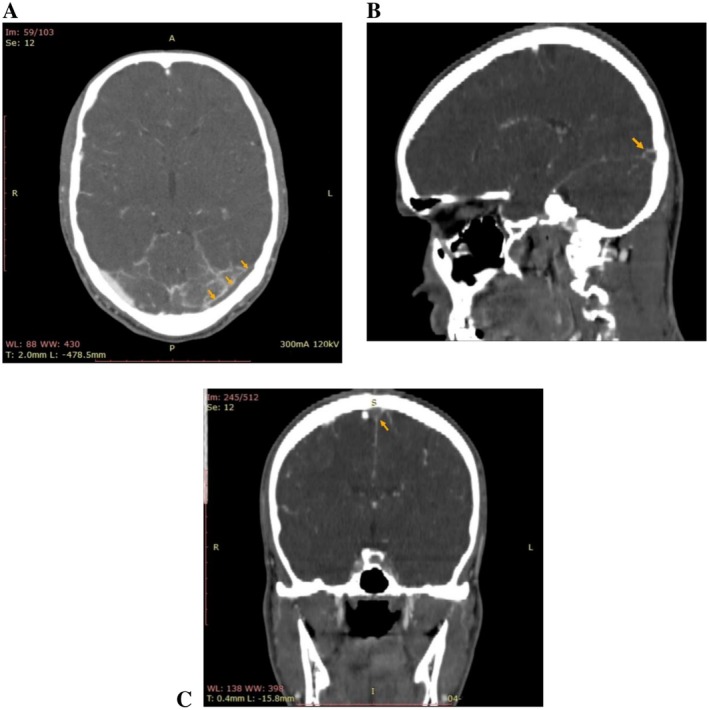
CT cerebral venography (selective images) showing hypodense filling defects, consistent with extensive dural venous sinus thrombosis. Axial view (A) demonstrating thrombosis in the left transverse sinus (yellow arrows). Sagittal view (B) demonstrating thrombosis at the confluence of sinuses (yellow arrow). Coronal view (C) demonstrating thrombosis in the superior sagittal sinus (yellow arrow), the classic ‘empty delta sign.’.
